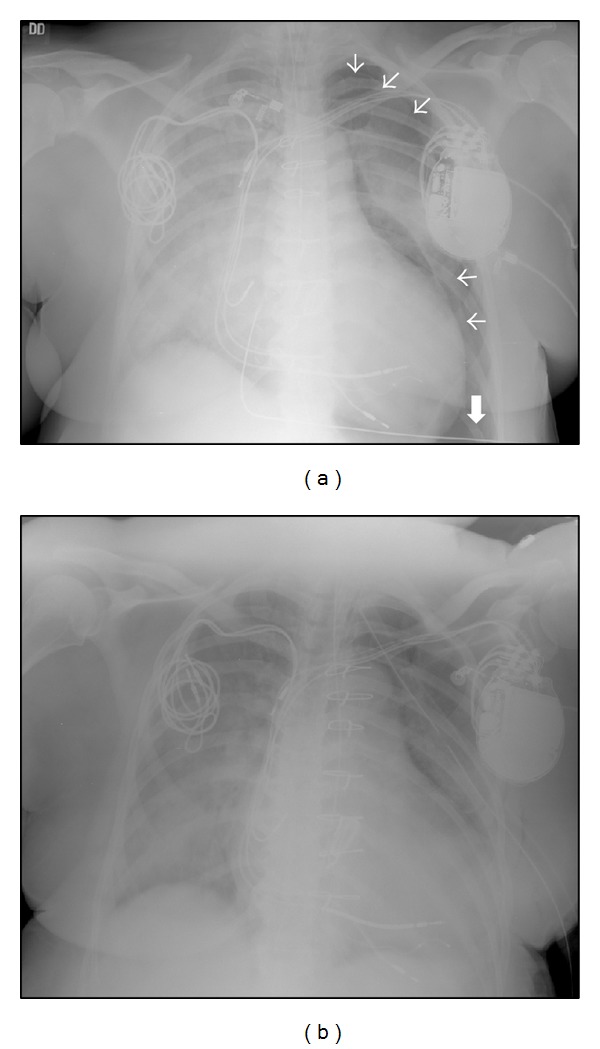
(a) Initial chest radiograph immediately following intubation revealing a large left-sided pneumothorax. Small arrows show the outline of the pneumothorax, partially obscured by the ICD. The large arrow shows depression of the left hemidiaphragm, which is a sign of tension pneumothorax. (b) Resolution of the pneumothorax after prompt chest tube placement.
